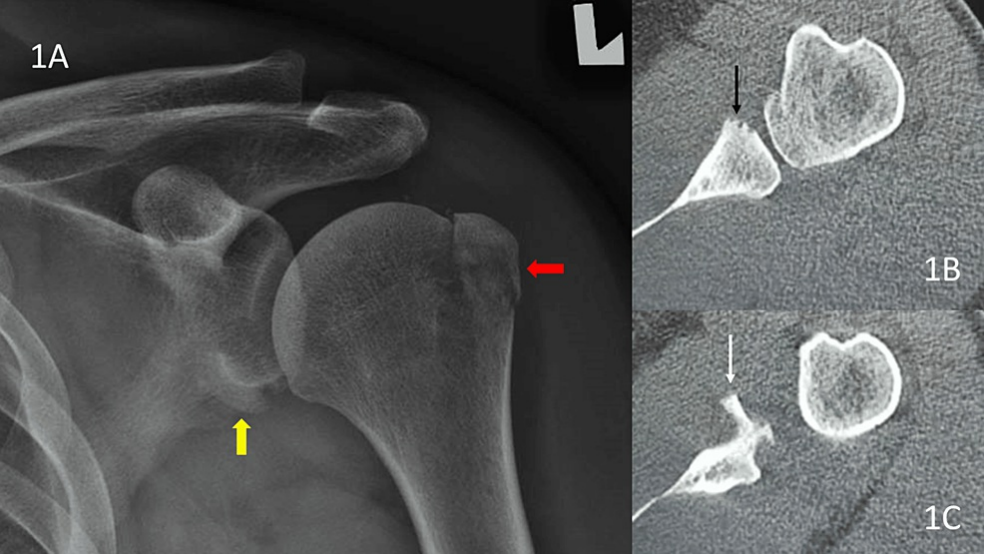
Plain anteroposterior (AP) radiograph and axial computed tomography (CT) images of the left shoulder. The greater tuberosity (GT) avulsion (red block arrow) with suspicious shadow at the glenoid neck (yellow block arrow) and the subsequent axial CT images showing antero-inferior glenoid defect (1B: black thin arrow) and a bony Bankart lesion displaced inferiorly (1C: white thin arrow).
